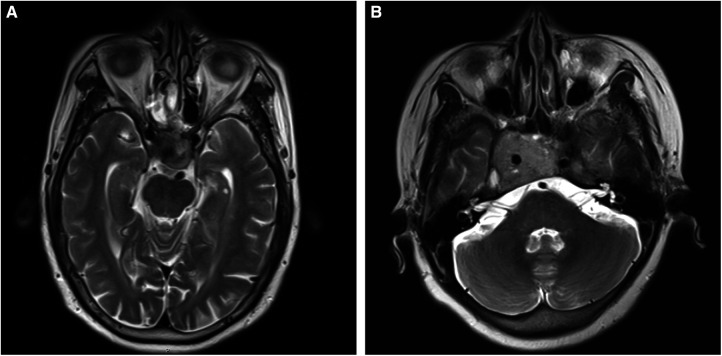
(A,B) T2-weighted images of nonfunctional pituitary adenomas with Knosp grades 3 and 4, respectively.
